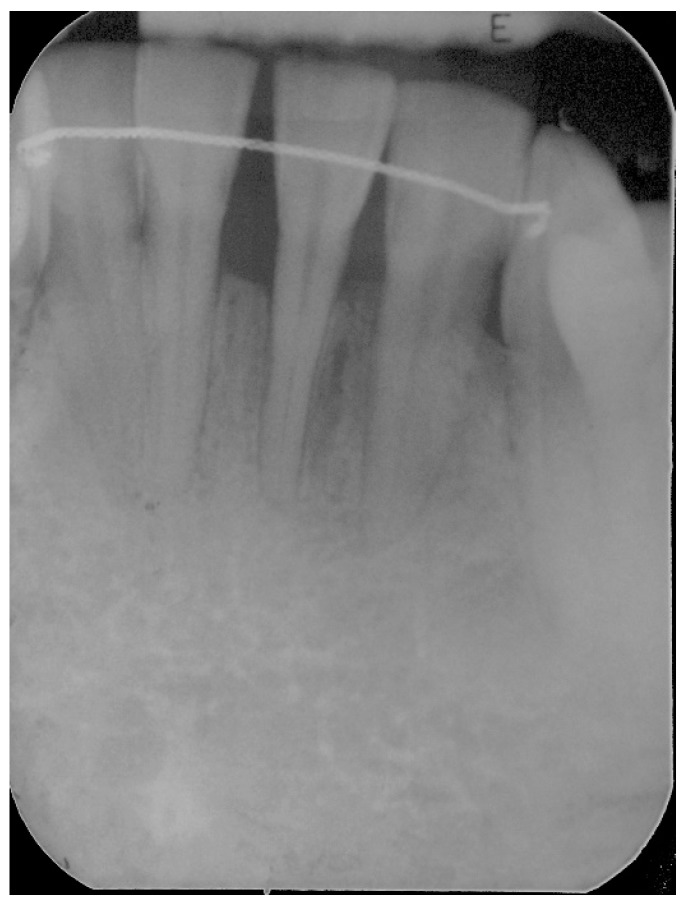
Two-year post-operative radiograph, stable results compared to the one-year situation are evident.
